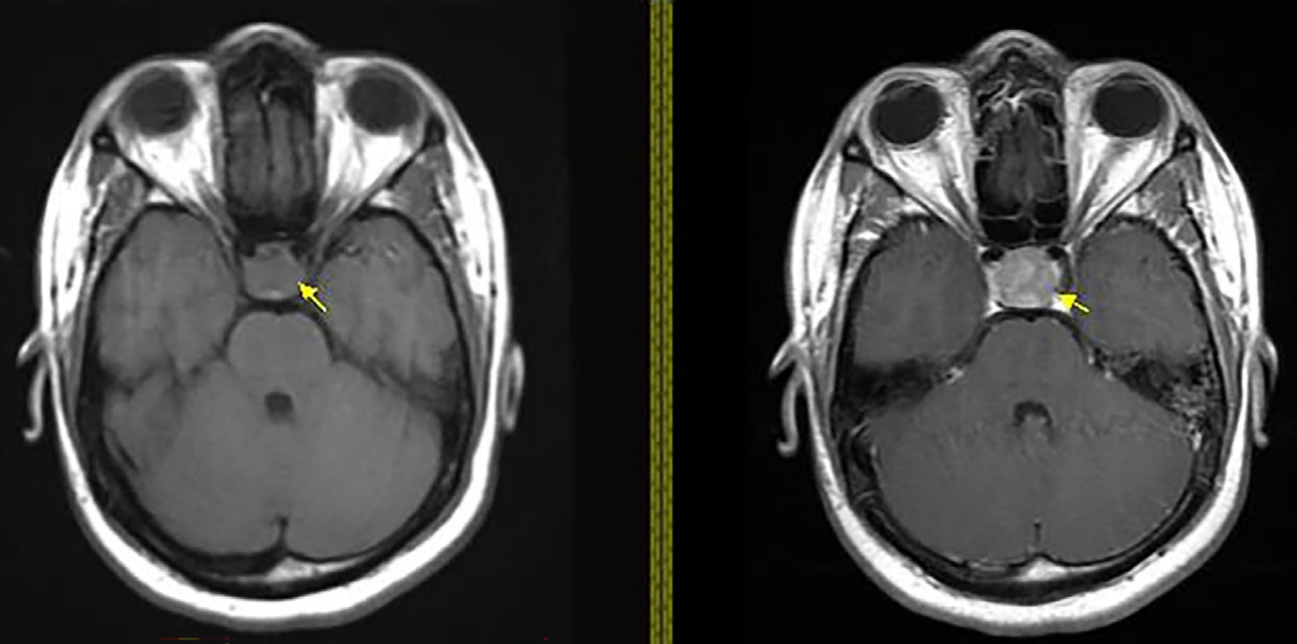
Initial Axial T1 MRI with (right) and without (left) contrast June 2021. Noncontrast image shows isointense lesion in the suprasellar region with small area posteriorly showing increased T1 signal. On contrast enhanced images this lesion shows heterogeneous enhancement most consistent with pituitary macroadenoma (yellow arrow).
